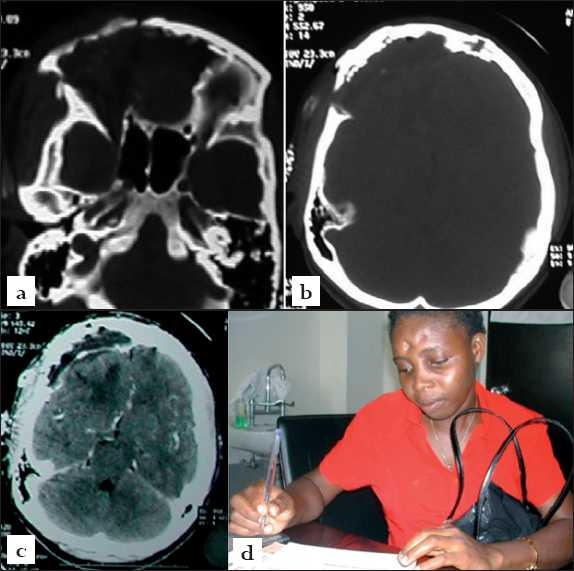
Postoperative images (a, b) Axial bone window images of the immediate postoperative cranial CT scanning confirming the operative complete lesionectomy, frontoethmoidectomy, unroofing of the right orbit and the reconstructed right orbital rim and the frontal basal skull convexity (c) good cerebral hemispheric decompression and (d) clinical picture of the patient 14 months postop
